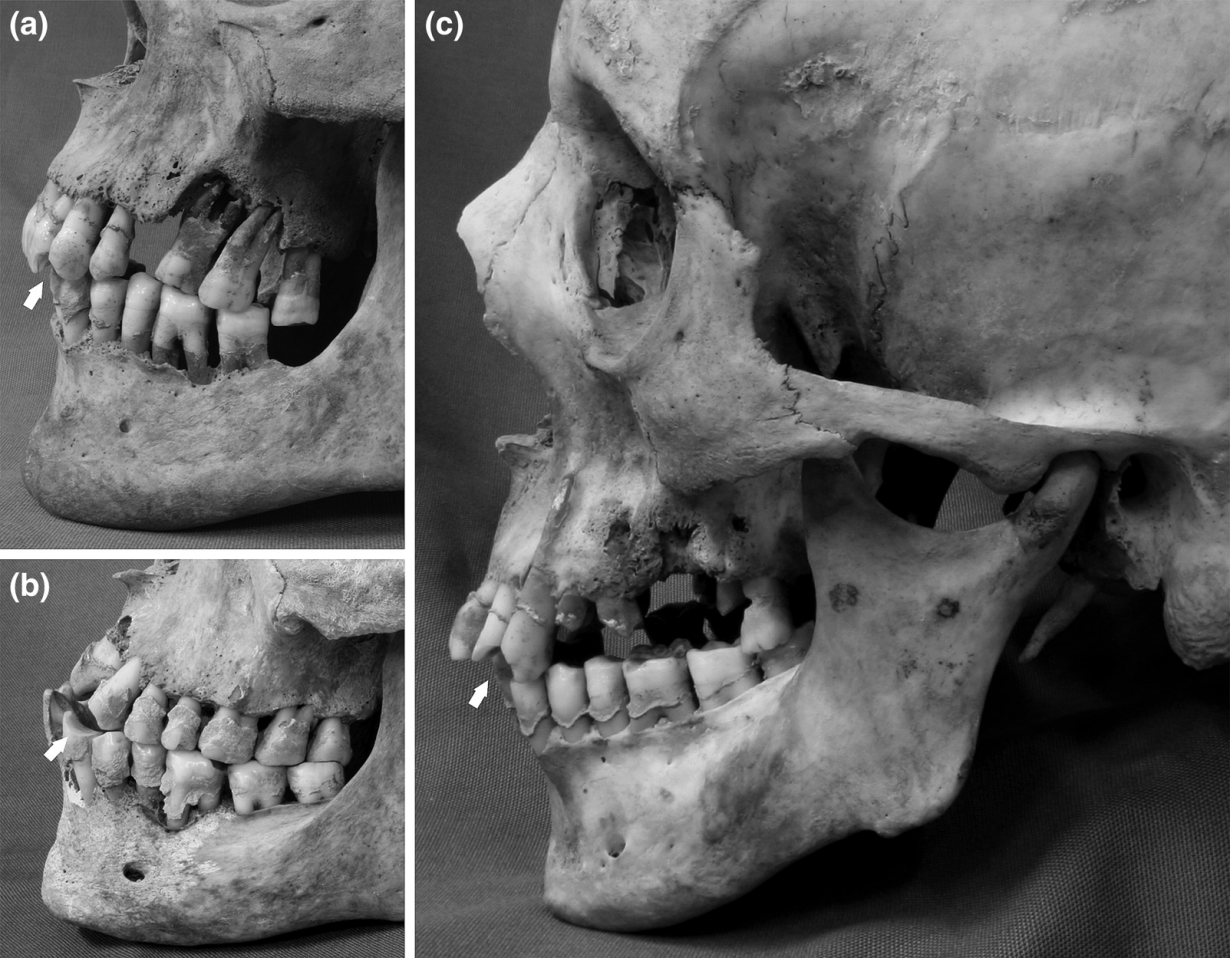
Examples of clay‐pipe facets (arrows) manifested in adult dentitions: (a) ≥46‐year‐old male (DCCLXV) with evidence of ante‐mortem tooth loss, dental caries, dental calculus and periodontal disease; (b) 36–45‐year‐old male (DCCLXXXV) with evidence of ante‐mortem tooth loss, dental calculus and ante‐mortem tooth loss; and (c) 26–35‐year old male (CCLXXXV) with evidence of ante‐mortem tooth loss, a periapical abscess, dental caries, dental calculus, and periodontal disease. Photo: Jonny Geber
